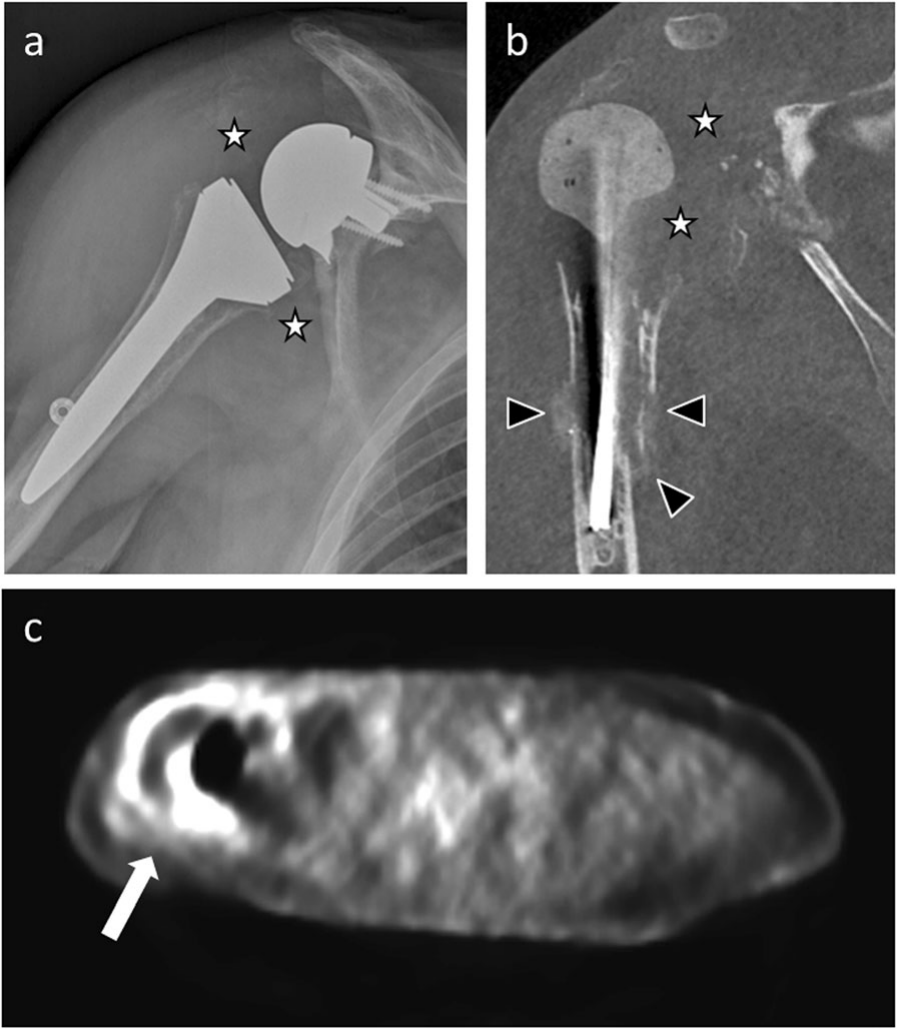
a Anteroposterior radiograph of a 73 years-old man with periprosthetic joint infection shows a soft-tissue thickening (white stars) around the reverse shoulder arthroplasty. b Coronal CT image of the same patient as in a, immediately after surgical revision and placement of a spacer, shows the same soft-tissue thickening (white stars), focal cortical losses, and periosteal appositions (arrowheads). c Axial FDG-PET image of the same patient as in a before surgery shows abnormal uptake around the prosthesis
